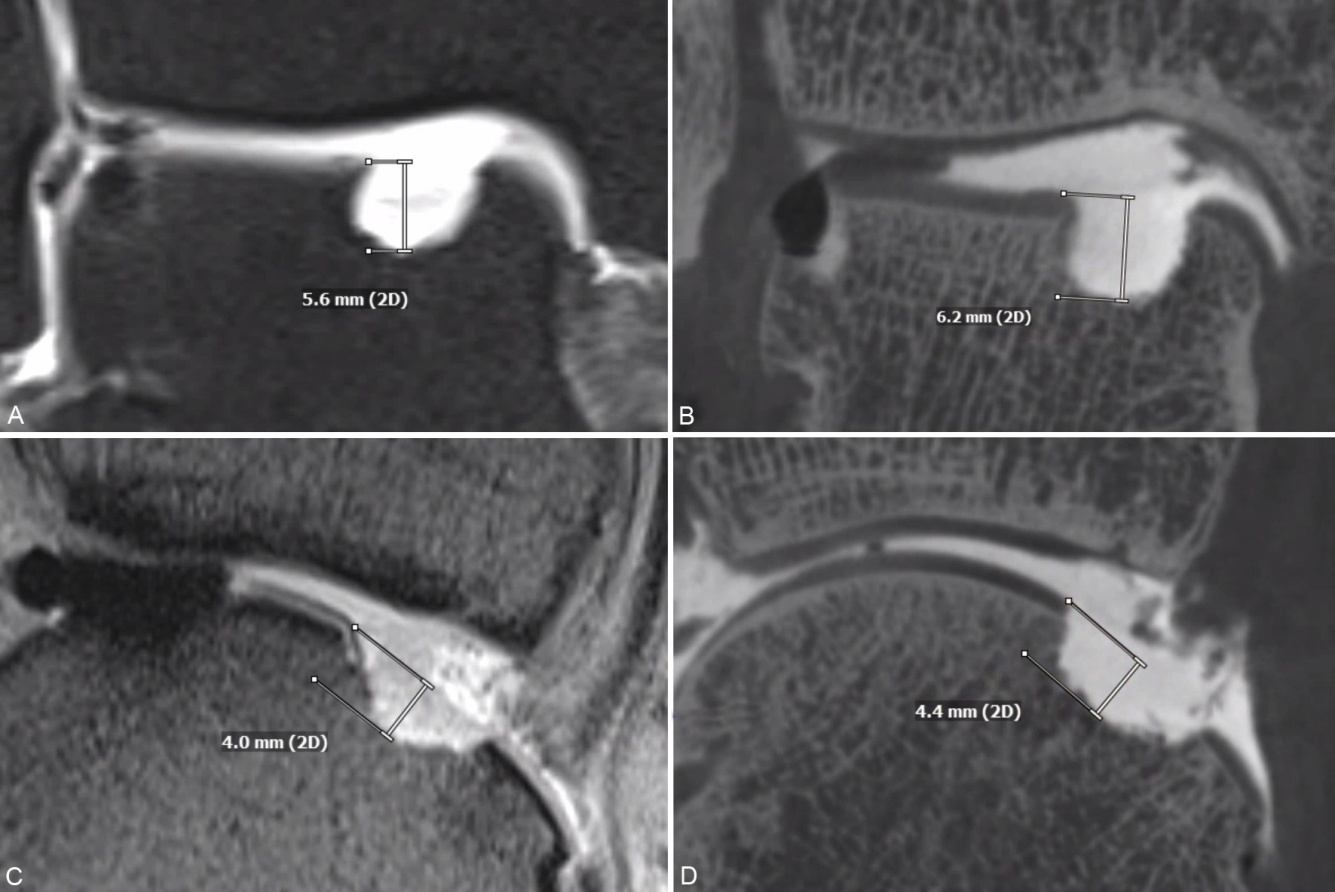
Different MR sequences and flat panel CT-arthrography measurements. Proton density weighted (PDw) coronal (A) and T1w sagittal (C) magnetic resonance arthrography (MR-A) sequences and the correspondingly reconstructed plane in FPCT-A images (B and D) of the same artificial defect. The marked depth measurements show larger values for the FPCT-A images.
